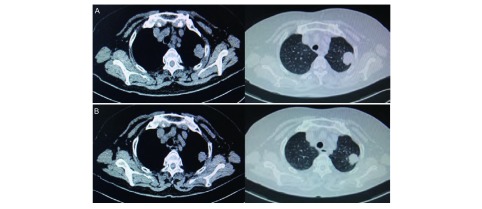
肺腺癌患者安某胸CT。A：服用阿帕替尼前；B：服用阿帕替尼3个月后。 The thoracic spiral CT of lung adenocarcinoma patient. A: Before using apatinib; B: Using apatinib three months later. CT: computed tomography.
